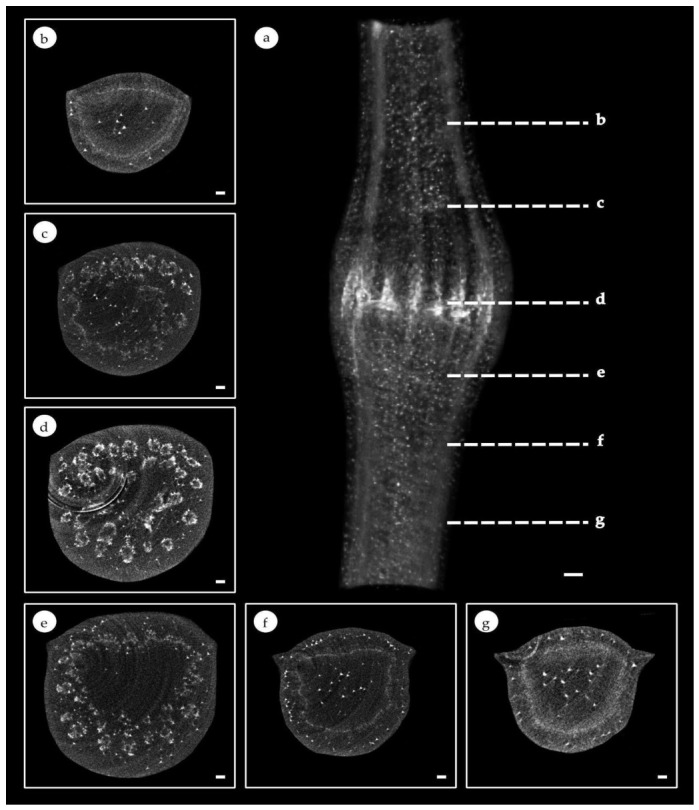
Micro–computed tomography images of *Erythrochiton brasiliensis*. (a) General view of the petiole showing trajectory of vascularization. (b–g) Sequential organization of the vascular system in the transverse section corresponding to regions indicated in (a). (b,c) Region above the swollen area with a closed vascular cylinder and no vascular plexus. (d,e) Swollen region, showing the lateral leaflet traces. (f,g) Region below the swollen area with a closed vascular cylinder similar to the region above the swollen area. Scale bars: (a) = 100 μm; (b–g) = 200 μm.
